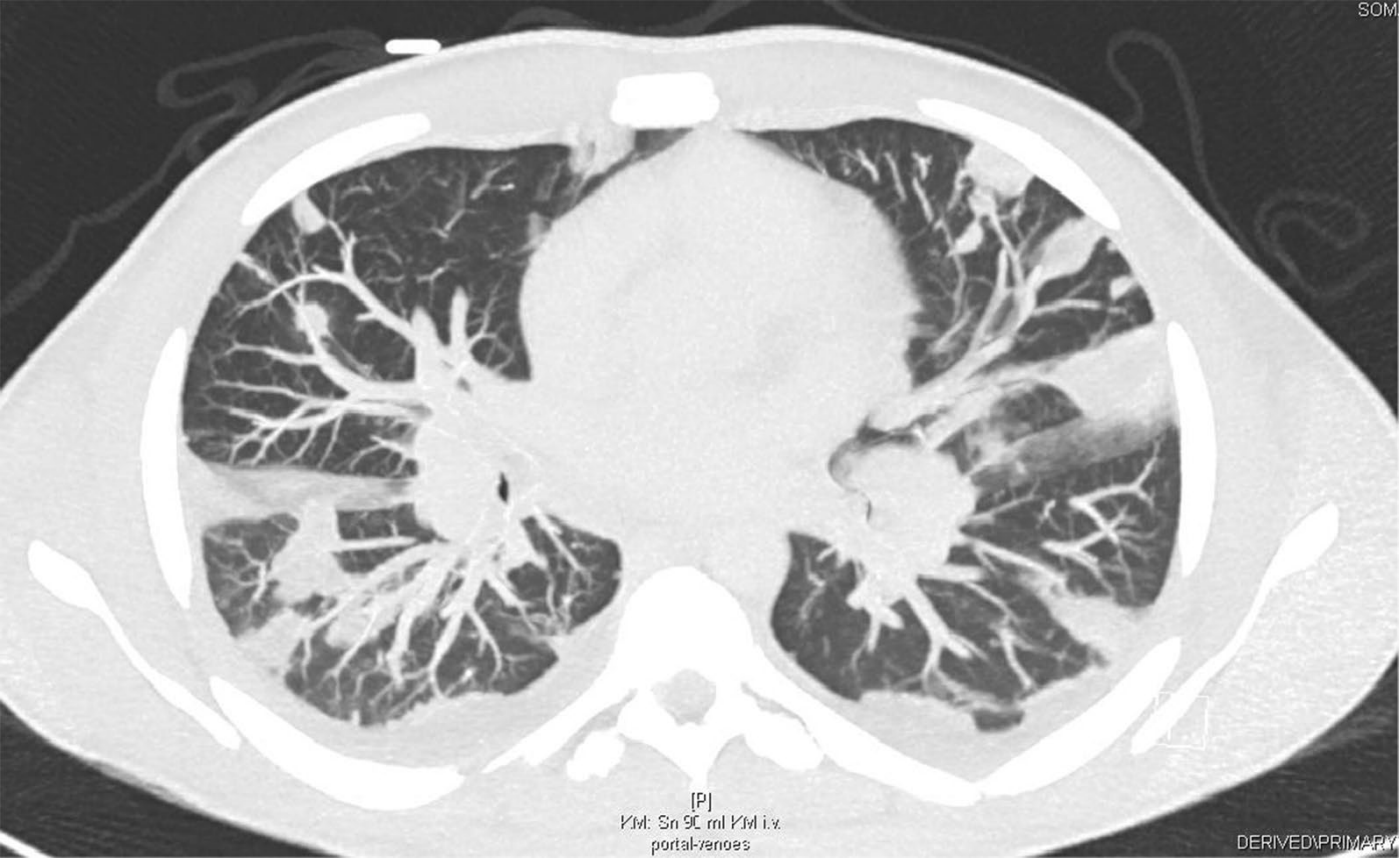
A thorax CT scan demonstrating bilateral pneumonic infiltrates, septic emboli, and pleural effusions
